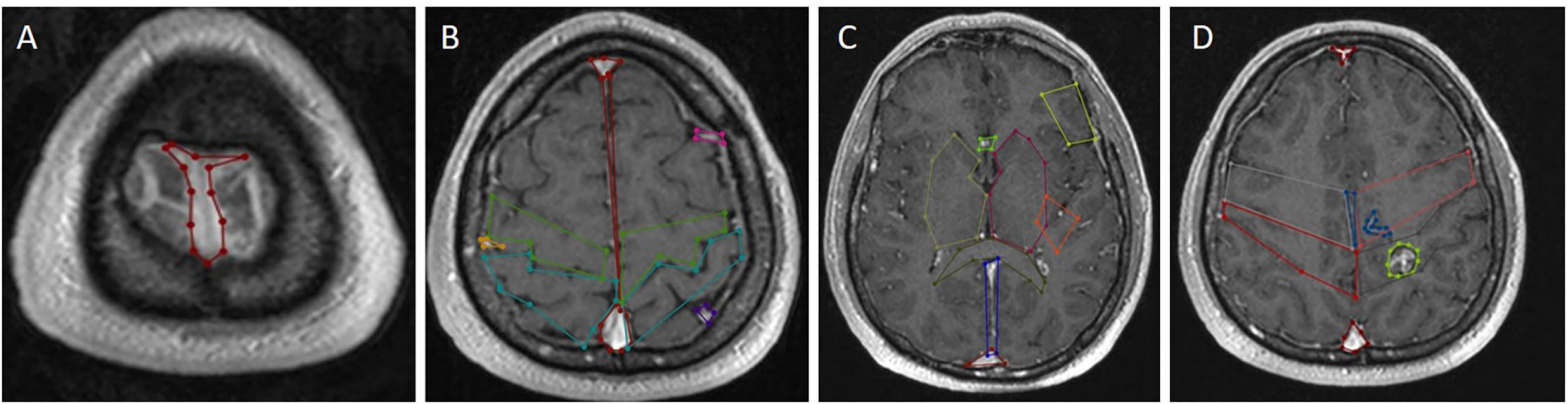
Labeling using contrast-enhanced T1 axial image of cranial MRI. (A) Superior sagittal sinus marked in red at the vertex's midline. (B) Superior sagittal sinus marked with red in the midline in the supraventricular area, precentral gyrus marked with green, postcentral gyrus marked with turquoise, superficial cortical veins marked with pink on the left and dark yellow on the right adjacent to the bilateral frontal lobes. (C) Right basal ganglia and thalamus marked with yellow in the right cerebral hemisphere at the ventricular level; left basal ganglia and thalamus marked with light red in the left cerebral hemisphere at the ventricular level, Broca's area in the left frontal lobe with light yellow, Wernicke's area posterior to Sylvian fissure marked with orange; The anterior cerebral arteries are marked in light green anteriorly in the midline, the corpus callosum splenium in green and the sinus rectus in blue in the midline posteriorly. (D) Right postcentral gyrus marked red, cavernom/tumor marked yellow-green, pericallosal artery marked blue on the midline and posterior inferior frontal artery marked blue.
